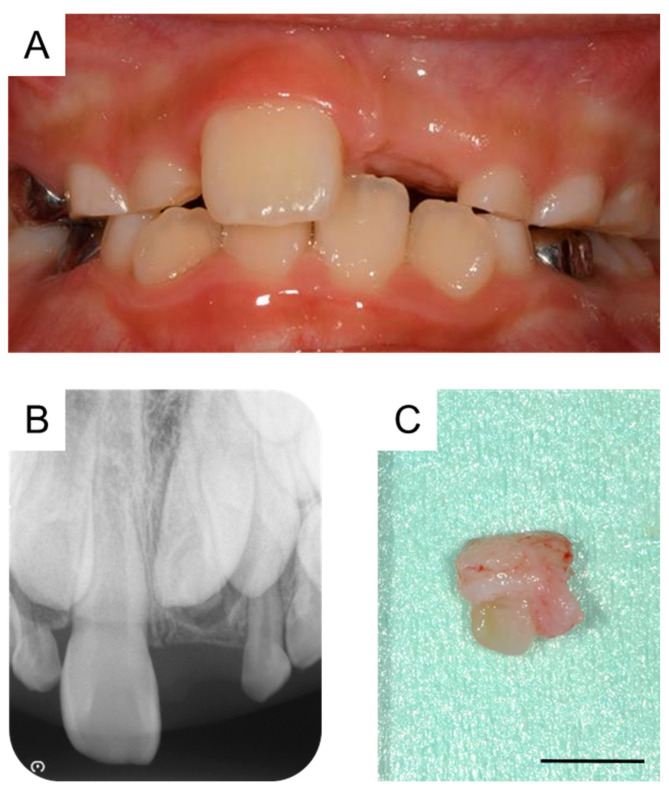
Extraction of the third supernumerary tooth. (A) Intraoral photograph at the age of 8 years and 2 months. (B) Periapical radiograph showing the third supernumerary tooth at the age of 8 years and 2 months. (C) Extracted calcified tissue, which was later diagnosed as the third supernumerary tooth. Scale bar: 5 mm.
